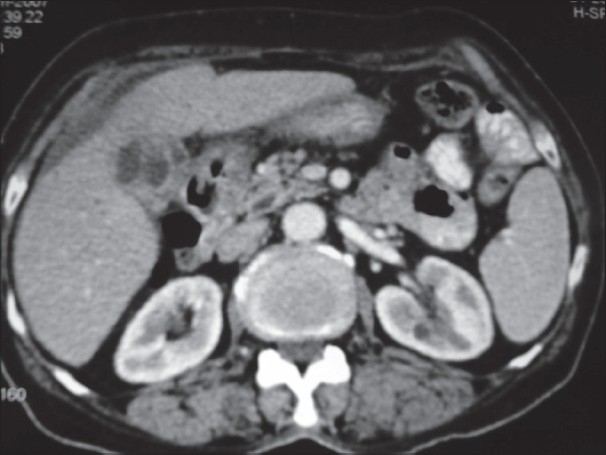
Computed tomography scan after rupture, which shows near-complete resolution of the large pseudocyst into the omental bursa with decompression of the biliary tree with a very small residual collection
